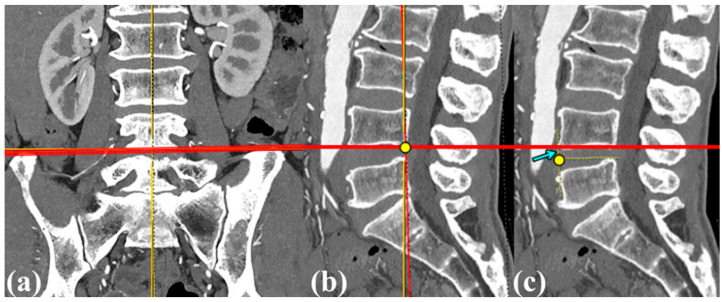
Measurement of the distance of the iliac crest in relation to the L4–5 disc space by computed tomography (CT). (a) The thick red line represents the connection between the highest points of both iliac crests in the CT coronal plane, with the midpoint of this line serving as a reference. (b) In the sagittal plane, the midpoint of the coronal plane’s red line is indicated by a yellow dot, which is then projected to create a horizontal red line. (c) The distance between the yellow dot marking the ventral side midpoint of the L4–5 disc space and the horizontal red line is measured as the distance between the iliac crest and the L4–5 disc space (light blue arrow).
